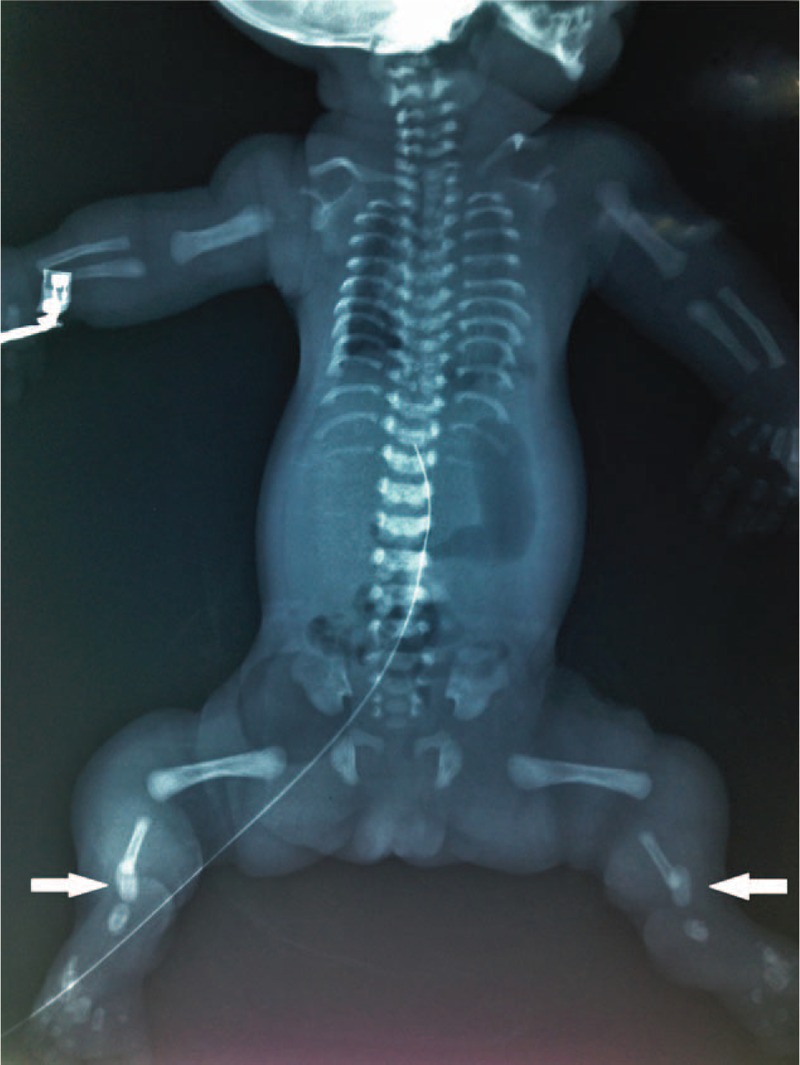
Radiograph of case 1 shows narrow thorax due to short and horizontally oriented ribs. The upper and lower limb long tubular bones are short. The pelvic appearance is normal. The metaphyseal ends of the long bones are rounded and there are extremely short ovoid tibiae (arrow).
